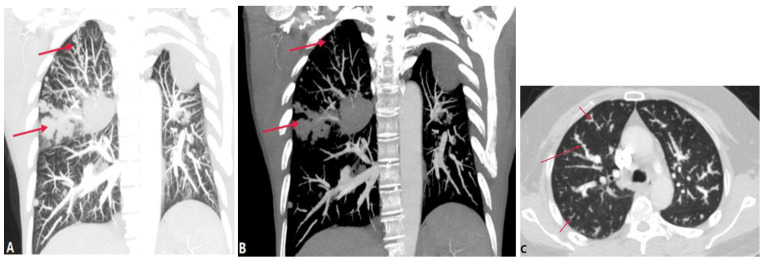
Tumor embolism and PTTM. (A): Coronal CT MPR image of a patient with right-lung cancer shows tumor emboli in the sub-segmental pulmonary arterial branches (arrows) and lumen occlusion. (B): Coronal CT scan image at the same level with soft tissue windowing shows the vascular dilatation and beading of sub-segmental arteries of the right pulmonary artery (arrows). (C): CT in another patient with a right atrium tumor shows tumor emboli with a tree-in-bud appearance within the secondary lobule arteries (short arrow) and beading of sub-segmental arteries (long arrow), which indicates PTTM.
